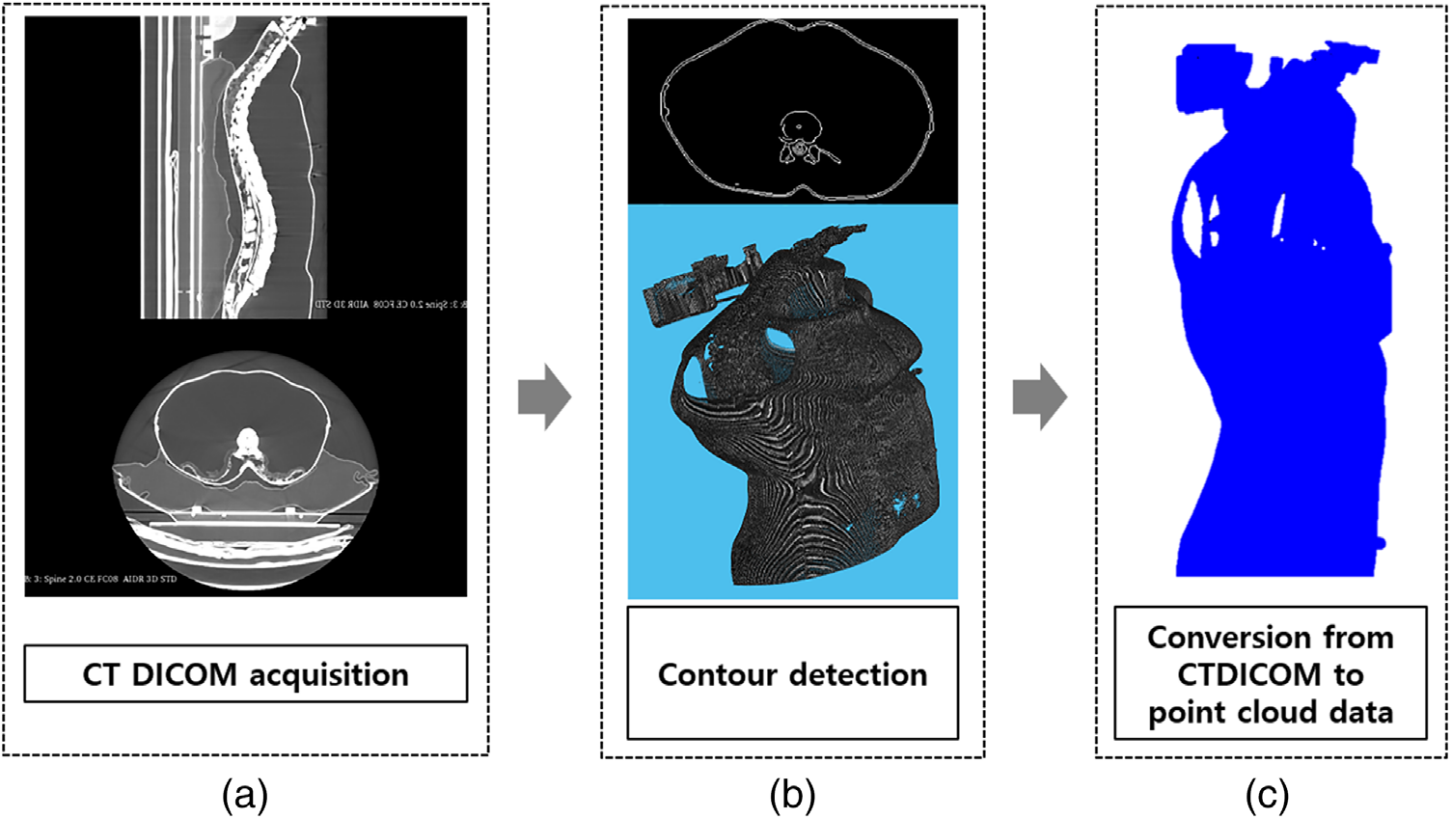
The conversion process of CT DICOM to 3D point cloud eventually obtained CT surface image
